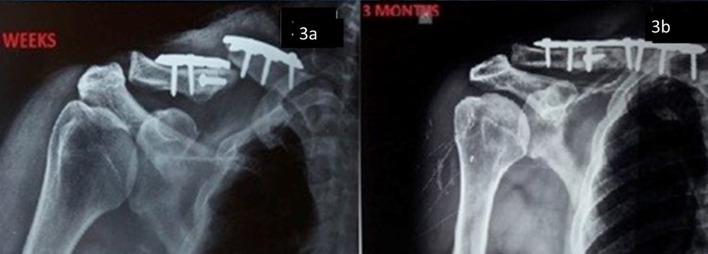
Complications after plating (a) Broken plate at 6 weeks, (b) postoperative x-ray 3 months after revision surgery.
